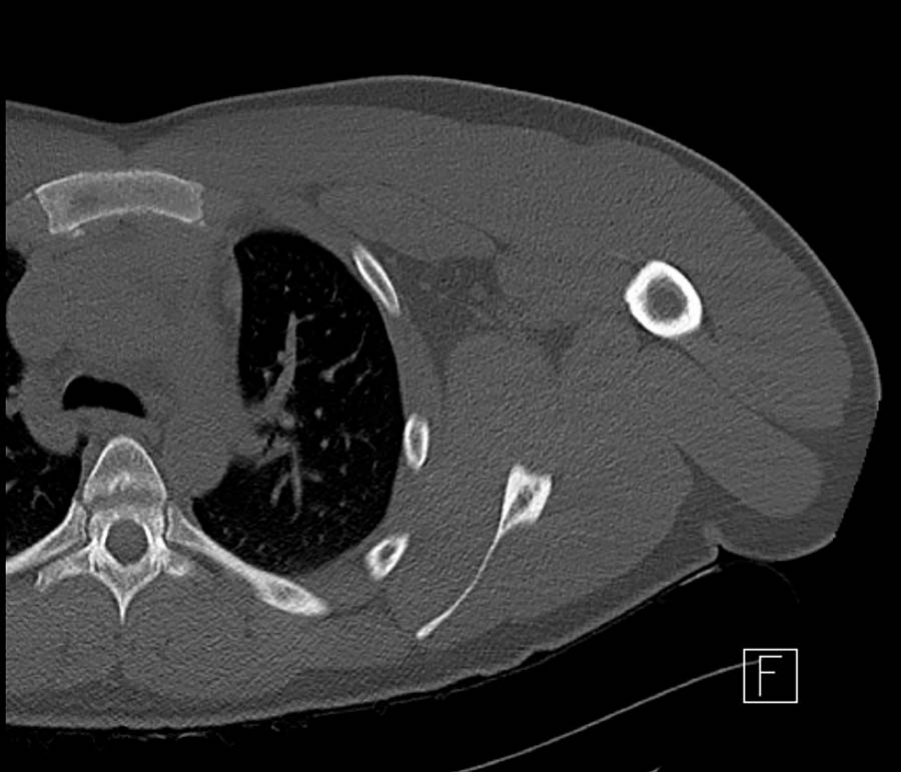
CT scan of left shoulder with no significant soft tissue or mediastinal abnormality.
